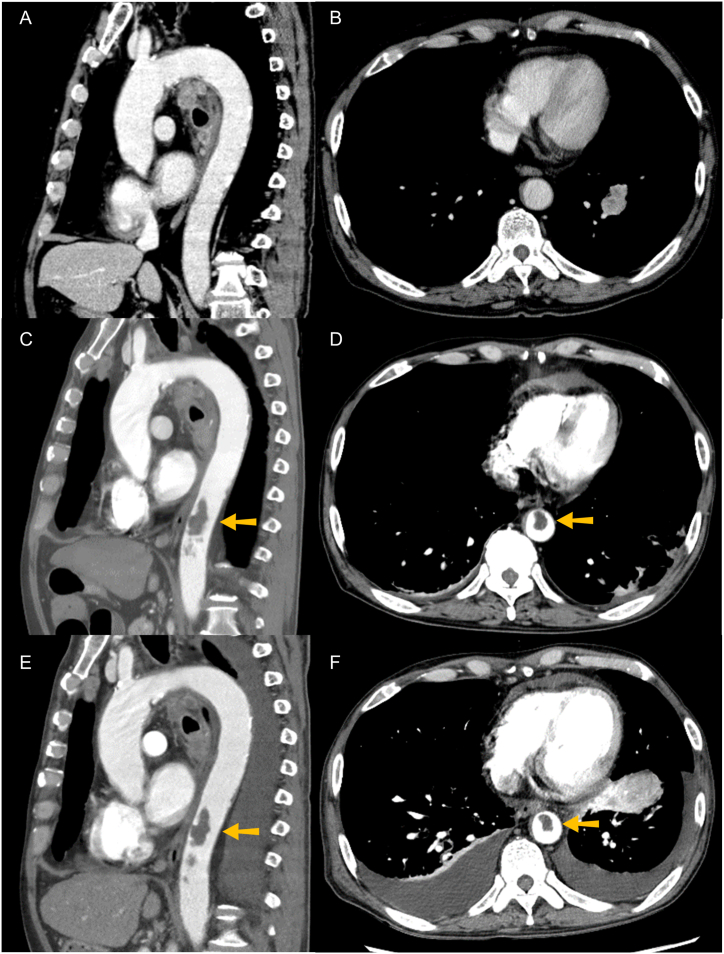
Enhanced computed tomography angiography images of aorta. There was no thrombus in the aorta at the lung cancer diagnosis (A, B). A massive thrombus was observed in the descending aorta (arrows) at the first day of admission (C, D). There was no obvious shrinkage in the aortic thrombus after 20-day anti-platelet therapy with aspirin and alprostadil (E, F).
